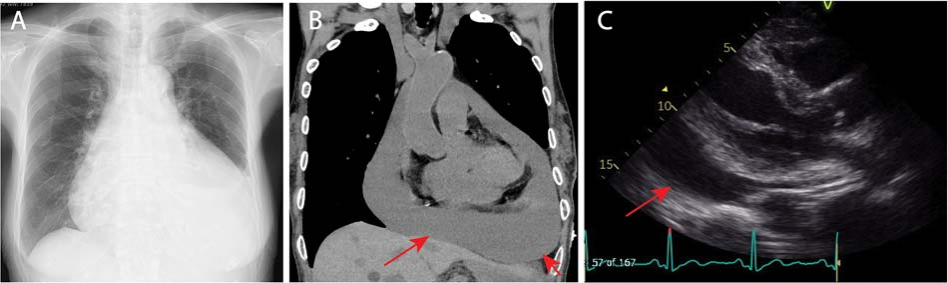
Preoperative images. (A) Preoperative chest X-ray. The cardiothoracic ratio was 0.70. (B) Preoperative computed tomography scan revealed massive cardiac effusion (arrow). (C) Preoperative echocardiogram revealed massive cardiac effusion (arrow).
